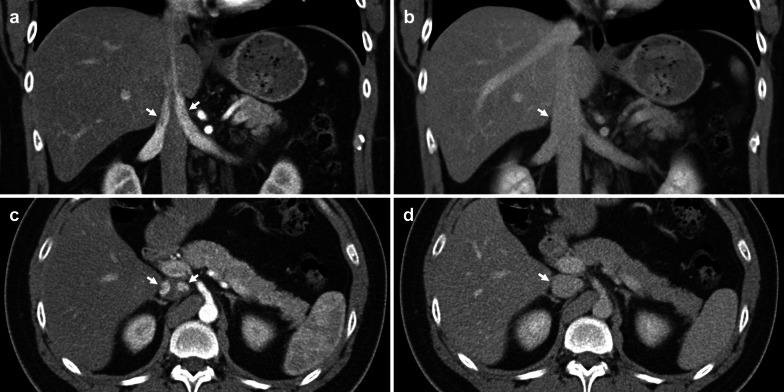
IVC pseudothrombus due to mixing artefact. a,c Arterial phase CT demonstrates an apparent filling defect in the IVC attributable to contrast-enhanced blood (arrows) draining from both renal veins and running alongside a column of non-enhanced blood from the lower extremities. b,d Coronal and axial CT images of the suprarenal IVC (arrows) show resolution of the filling defect in portal venous phase
